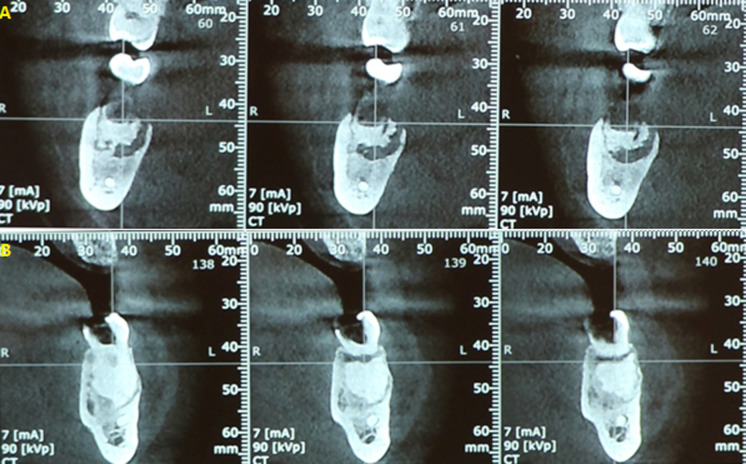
Cone Beam mandibulaire; A) calcification appendue à l´apex de la 36 cariée; B) calcification au niveau du site de la 47 extraite
